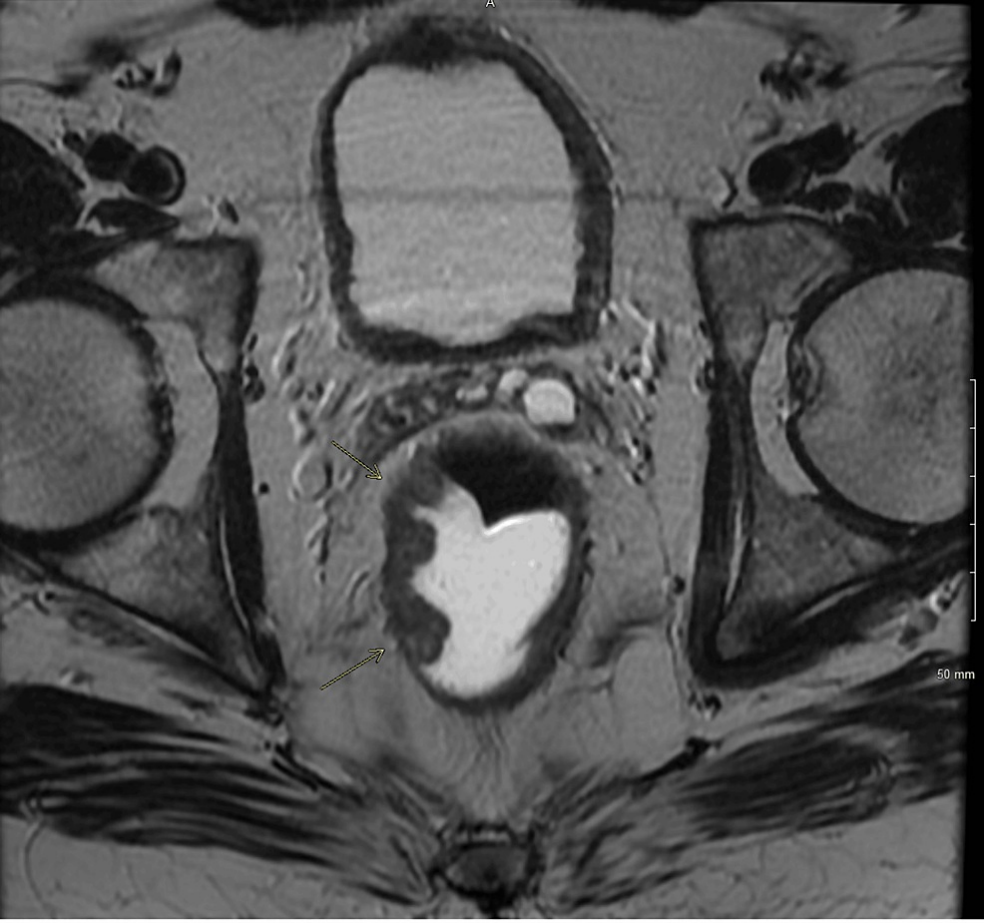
Axial MRI of the rectum with contrast revealing a 3.3 cm polypoid lesion centered in the mid-rectum. The lesion extends to less than 1 mm beyond the muscularis propria. The distance to mesorectal fascia (circumferential resection margin/CRM): 1.00 cm.
